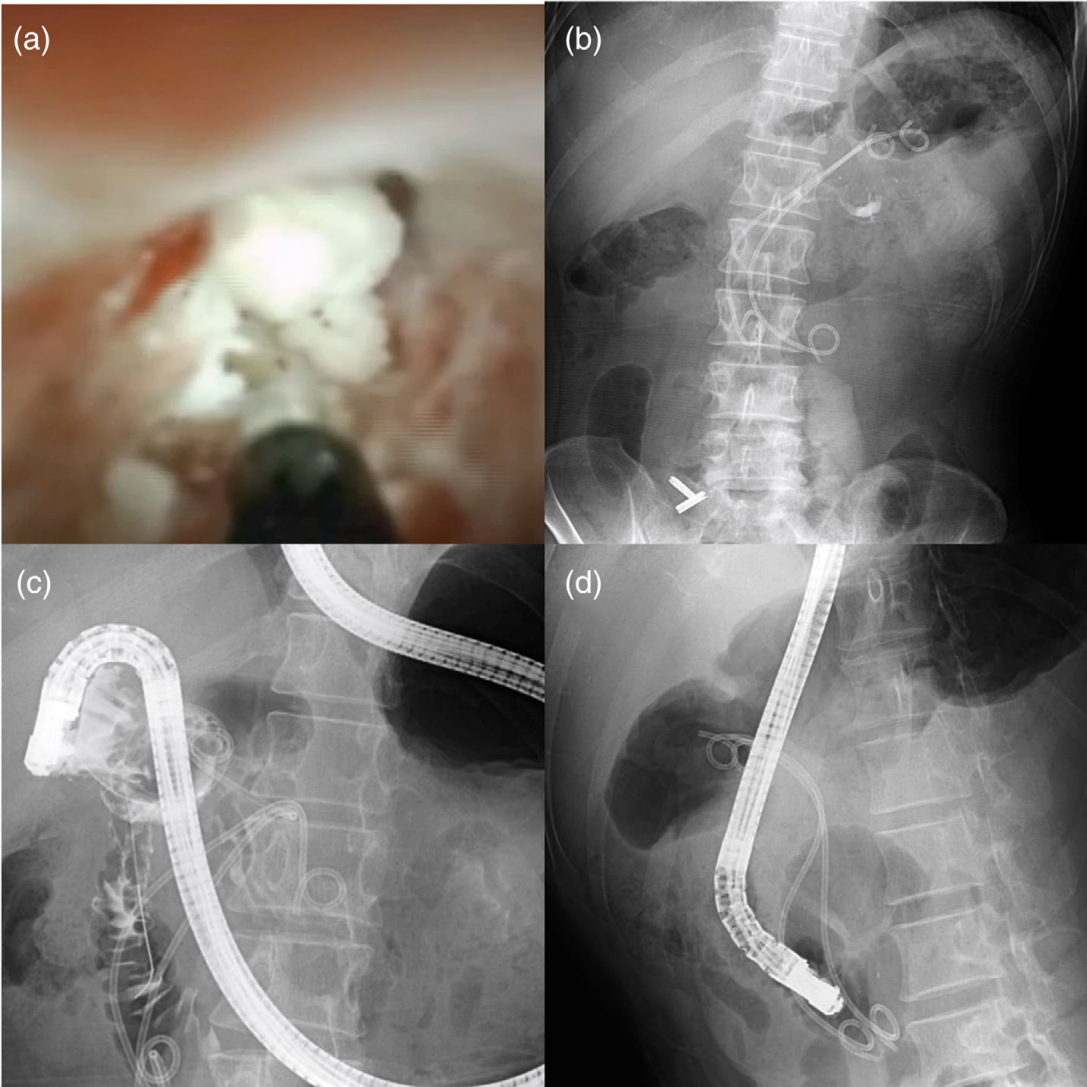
Electrohydraulic lithotripsy with pancreatoscope from endoscopic ultrasonography‐guided pancreaticogastrostomy (route and duodenoscope passage. (a) Electrohydraulic lithotripsy under a pancreatoscope (SpyScope DS; Boston Scientific, Marlborough, MA, USA) was performed for pancreatic stone treatment after dilation of the EUS‐PGS anastomosis. (b) After the pancreatic stone was crushed completely, two 7‐Fr × 12‐cm pigtail stents were placed in each Wirsung's and Santorini's duct. (c) At 1 month after the EUS‐PGS, the duodenal stricture was difficult to pass the duodenal stenosis. (d) At 7 months after the endoscopic ultrasonography‐guided pancreaticogastrostomy, a duodenoscope could pass the duodenal stenosis
